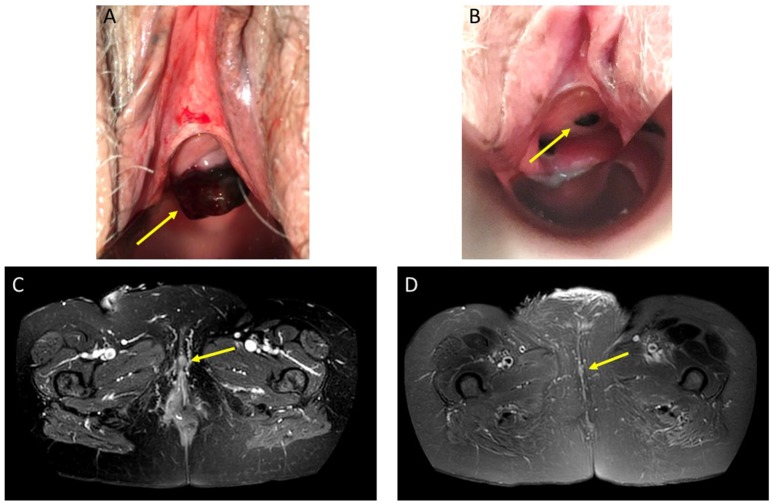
Clinical and MR image of intravaginal mucosal metastasis of a malignant melanoma of the urethra. A/B: Visual appraisal with speculum. (A) Target lesion before injection of T-VEC. Baseline image shows a pigmented ulcerated mucosal tumor (arrow). (B) Target lesion after seven injections of T-VEC: partial response with substantial regression of the injected mucosal metastasis (arrow) and cessation of intravaginal bleeding. C/D MR image. (C) Transverse T2-weighted fat suppressed MR image shows labial metastasis (arrow) before injection of T-VEC. (D) Transverse T2-weighted fat suppressed MR image 2 months upon T-VEC therapy shows complete disappearance of labial metastasis (arrow indicating the original location).
